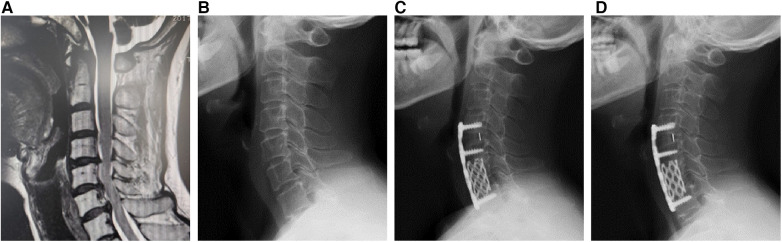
Female, 50-year-old, underwent one-level anterior cervical discectomy and fusion (ACDF) and one-level anterior cervical corpectomy and fusion (ACCF) surgery. (A) The pre-operational MRI, (B) the x-ray of pre-operation, (C) operation after 3 months, and (D) the final follow-up.
